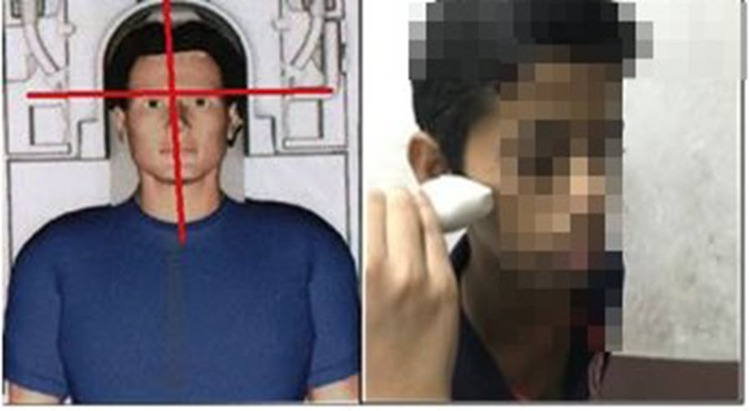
Patient position for MRI and ultrasound imaging
